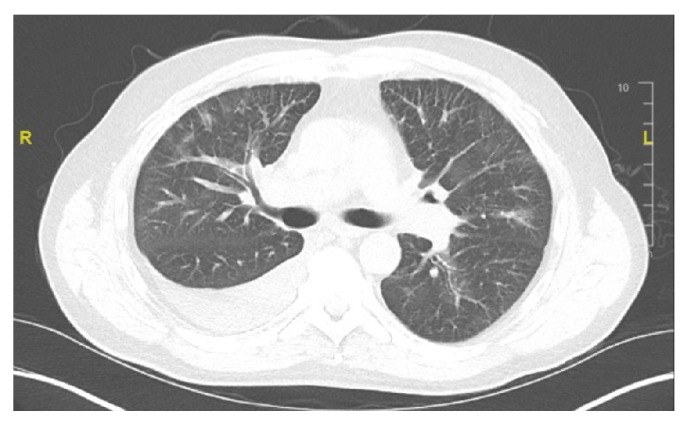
Repeat CT thorax without contrast showing moderate-sized right pleural effusion with right lower lobe atelectasis.
